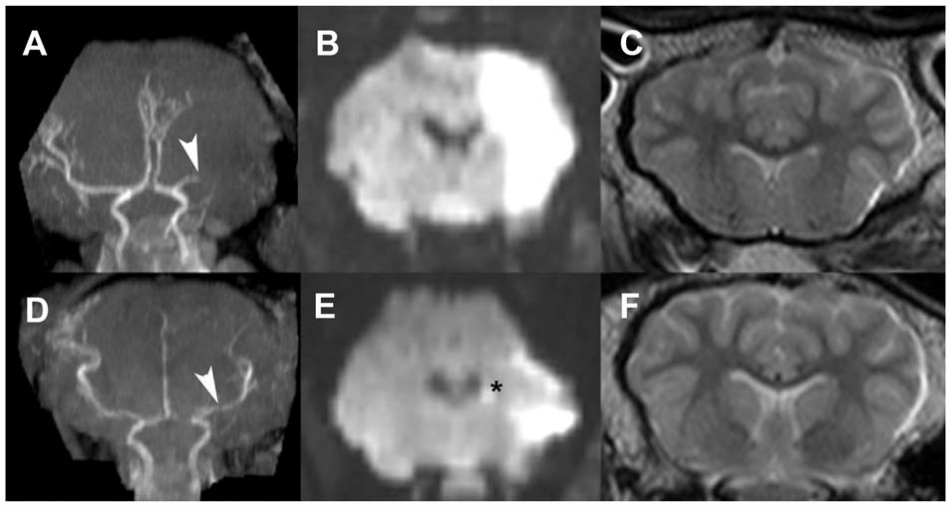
MRI at 4 hours after MCAO, coronal orientation. Permanent MCAO MRA (A), DWI (B) and T2 weighted imaging (C). Temporary MCAO with reperfusion MRA (D), DWI (E) and T2 weighted imaging (F). Arrowhead on MRA indicates site of arterial occlusion. Asterisk indicates restricted diffusion at caudate head after temporary MCAO. DWI, diffusion weighted imaging; MCAO, middle cerebral artery occlusion; MRA, magnetic resonance angiogram; MRI, magnetic resonance imaging.
